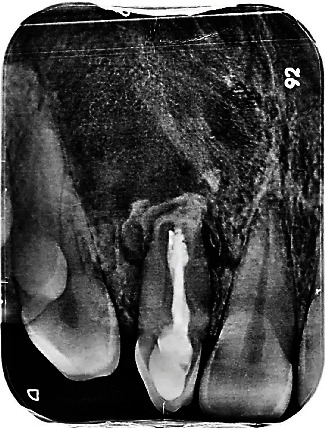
18-month follow-up radiograph showing signs of bone neoformation.
